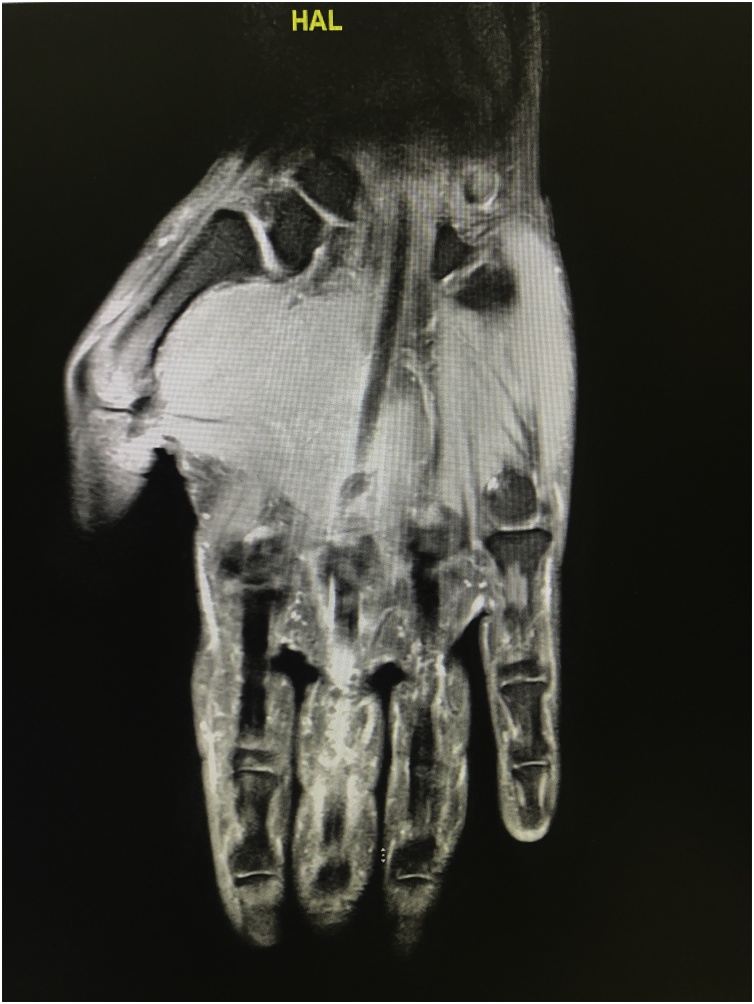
MRI hand showing discontinuty of FDS tendon.
